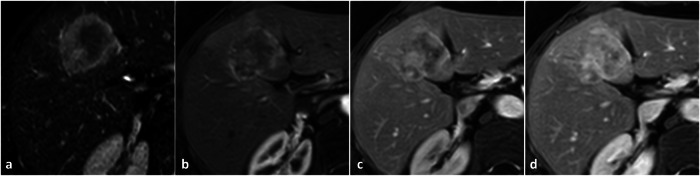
Intrahepatic cholangiocarcinoma (biopsy-proven and resected). The lesion shows heterogeneous signal intensity on T2-weighted images (a) with peripheral hyperintensity associated with central hypointensity. During the dynamic study, peripheral rim enhancement is appreciable in the arterial phase (b), followed by centripetal filling in the portal venous (c) and equilibrium (d) phase
